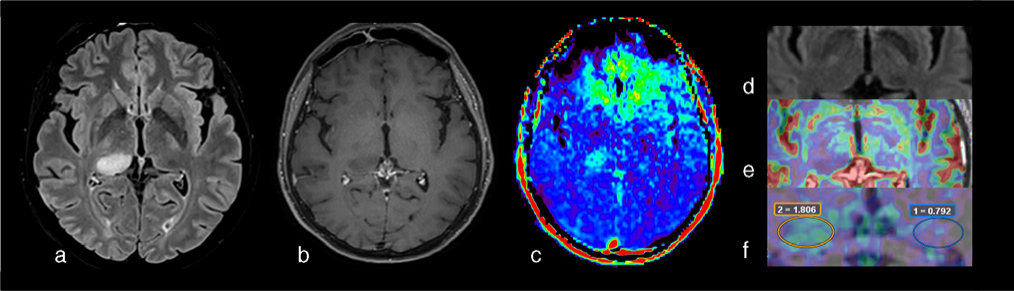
Example of a molecular defined glioblastoma (astrocytoma with *EGFR* gene amplification, therefore glioblastoma IDH-wildtype, WHO grade 4) displaying a well-limited lesion without mass effect, contrast enhancement, diffusion restriction nor hyperperfusion but with high APTw values. (a) 3D FLAIR. (b) 3D T1 w after injection. (c) APTw map. (d) axial diffusion, b value of 1000 s/mm^2^. (e) rCBV map after leakage correction superposed to T1w contrast injected sequence. (f) APTw map superposed to 3D T1w injected sequence. APTw, amide proton transfer-weighted; FLAIR, fluid attenuated inversion recovery; rCBV, relative cerebral brain volume.
